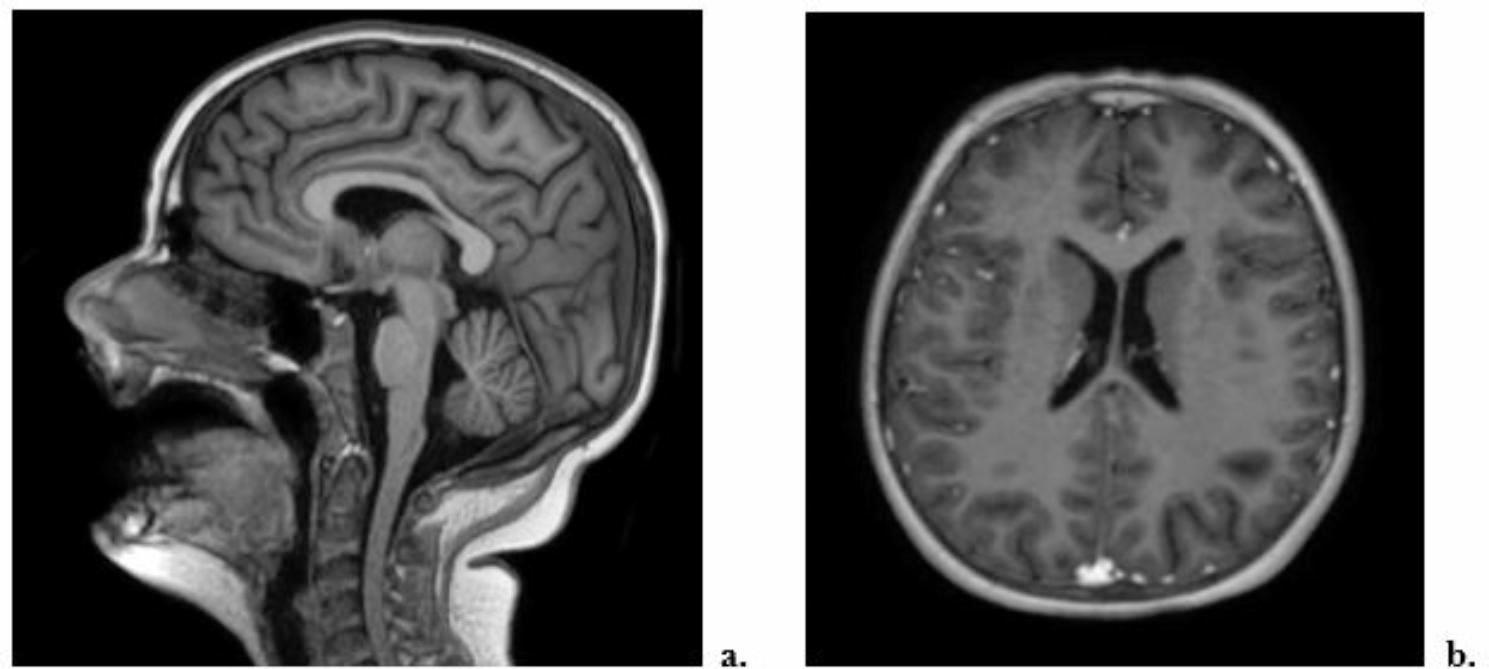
Brain MRI: a. sagittal view; b: axial view
